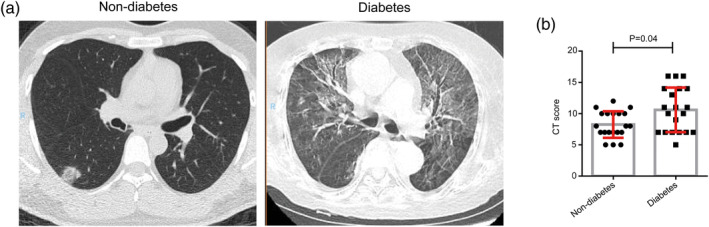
CT results of the patients with diabetes and patients without diabetes. A. The representative CT images of the patients with diabetes and patients without diabetes. B, The CT score of the patients with diabetes and patients without diabetes. *P* < .05 was considered statistically significant. CT, computed tomography
